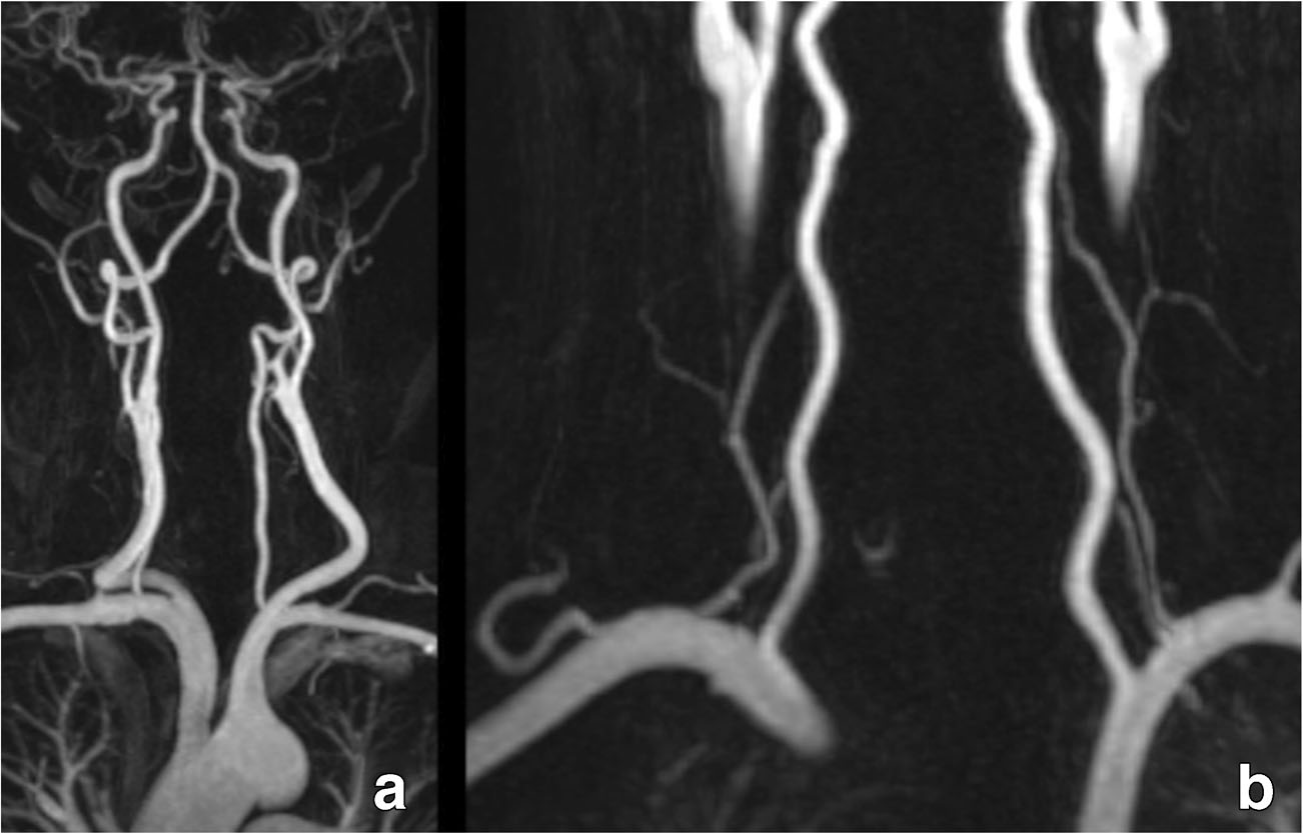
Contrast-enhanced gradient echo T1-weighted MR angiography of the cervical arteries. Conversely to CT angiography, MIP-images in MR angiography provide direct visualization of the whole trajectory of the carotid- and cervical arteries without bone- or other soft tissue superposition (a). This allows excellent visualization of segments which are near bones at the base of the neck, such as the origin of the vertebral arteries (b). MIP, maximum intensity projection
